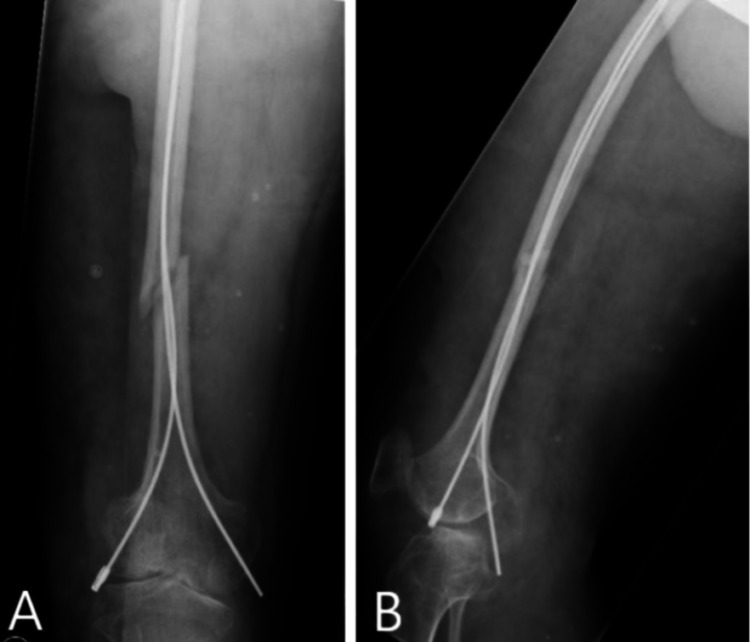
Radiographs immediately after reduction of fracture showing anteroposterior (A) and lateral (B) views
